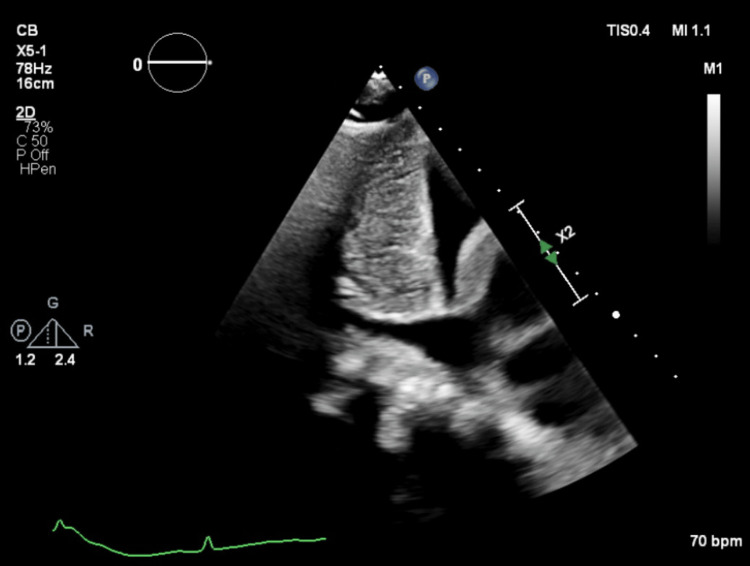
Subcostal two-dimensional echocardiographic view demonstrating a large pericardial effusion and small collapsible inferior vena cava.
